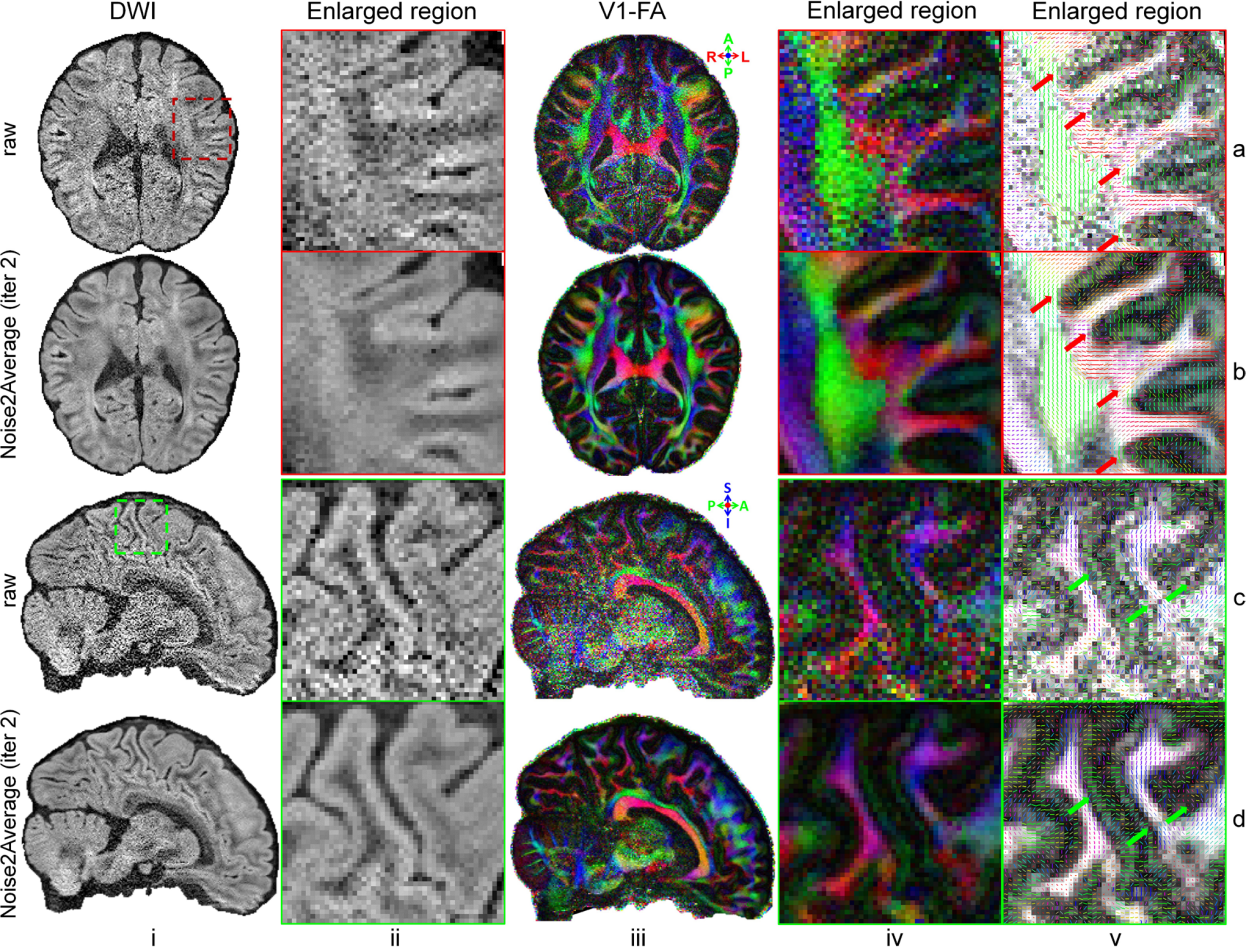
Noise2Average results of ultrahigh resolution diffusion data. Exemplary axial and sagittal image slices from the average of diffusion-weighted image (DWI) volumes acquired with anterior-posterior (AP) and PA phase encoding directions along diffusion-encoding direction [-0.96, -0.06, 0.28] (a, i and c, i) and Noise2Average-denoised image volume at iteration 2 (b, i and d, i) of a representative subject from MGH gSlider-SMS diffusion MRI data are displayed, along with enlarged views (ii) of regions of interest of cortical gyri (red box in a, i and green box in c, i). The DTI primary eigenvector (V1) encoded fractional anisotropy (FA) maps (red: left-right; green: anterior-posterior; blue: superior-inferior) from the raw and Noise2Average-denoised data (iii and iv) are displayed, with V1 rendered as color-encoded sticks superimposed on FA maps (v). The arrowheads highlight U-fibers in the superficial white matter (red, a, b, v) and fiber crossings at the gray-white junction (green, c, d, v).
